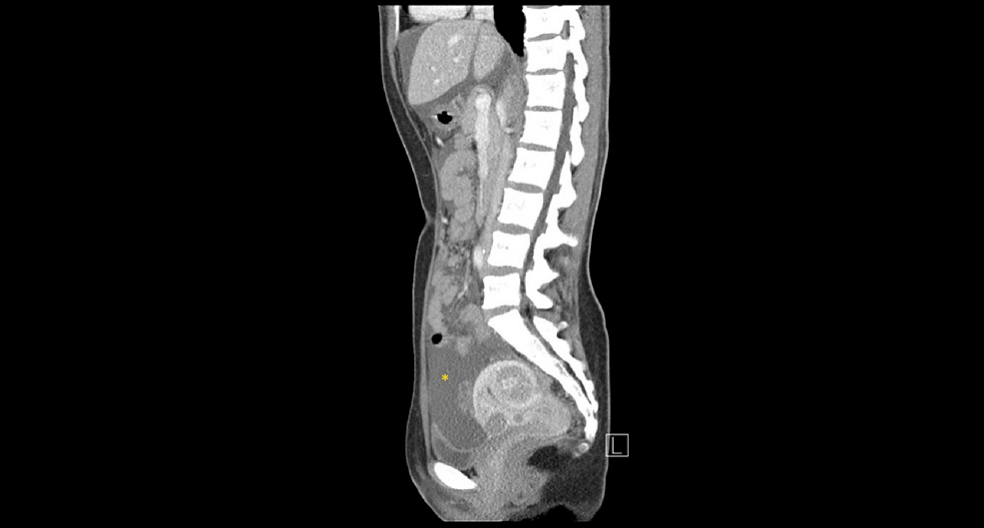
Abdominal CT scan – sagittal view showing massive ascites (asterisk) CT: computed tomography
